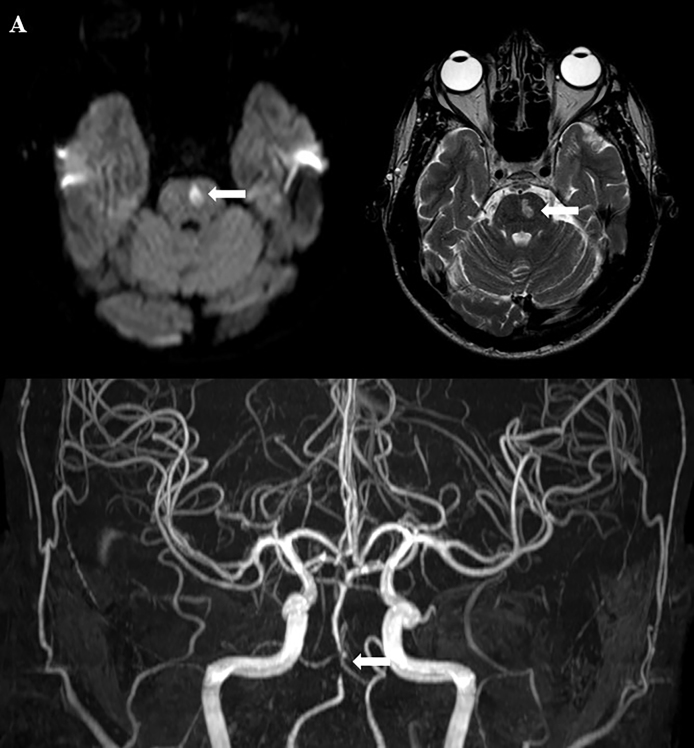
Axial DWI and T2-weighted MRI showing left hemipons infarction (arrows, A and B). Three-dimensional time-of-flight magnetic resonance angiography showing significant stenosis of the basilar artery (arrow, C).
